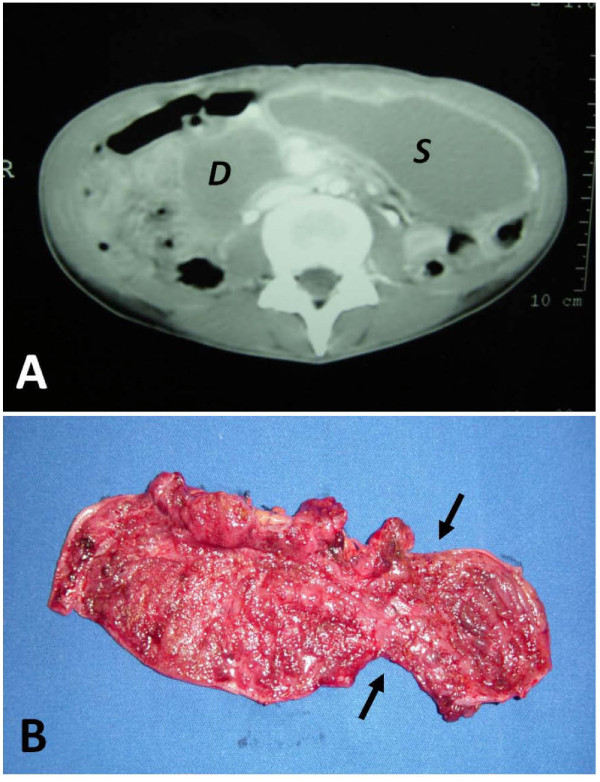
(A) Abdominal CT scan showing a large dilation of stomach (*S*) and duodenum (*D*). (B) Severe inflammation, mucosal hemorrhage and focal ulcerations of duodenum and proximal jejunum. Black arrows show the point of obstruction.
